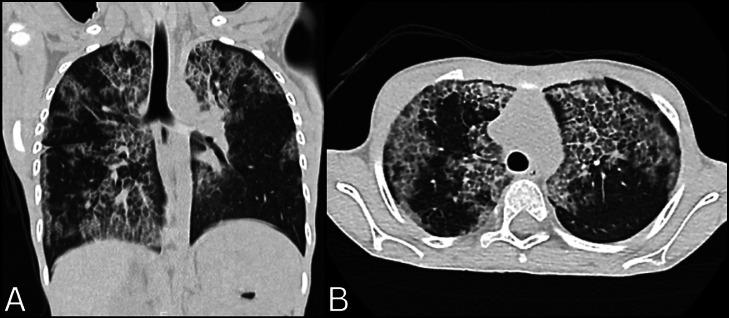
High-resolution computed tomography (HRCT) (A) coronal and (B) axial view of the chest showing large patchy areas of ground glass opacities with interlobular septal thickening involving all lobes of bilateral lung parenchyma, resulting in a crazy-paving pattern.
